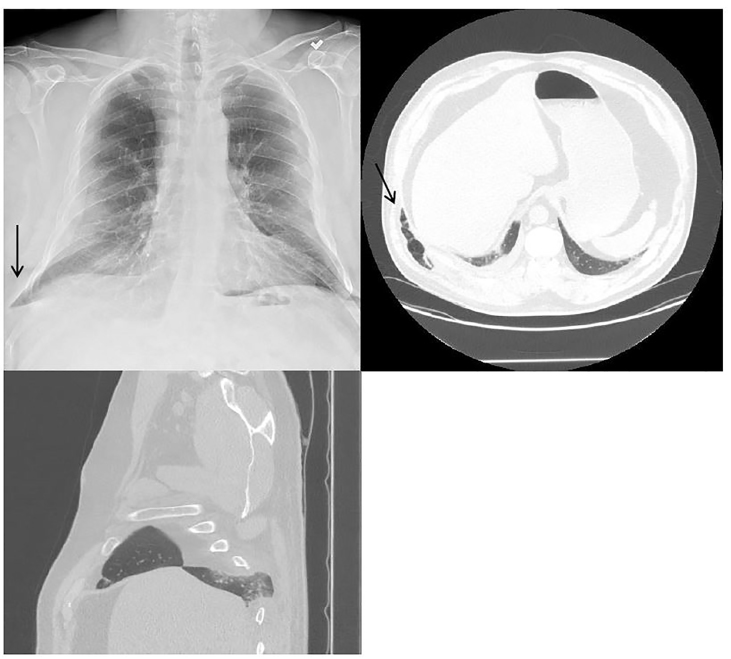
Computed tomography and chest raimgdiograph demonstrating right-sided intercostal lung herniation (arrow).
